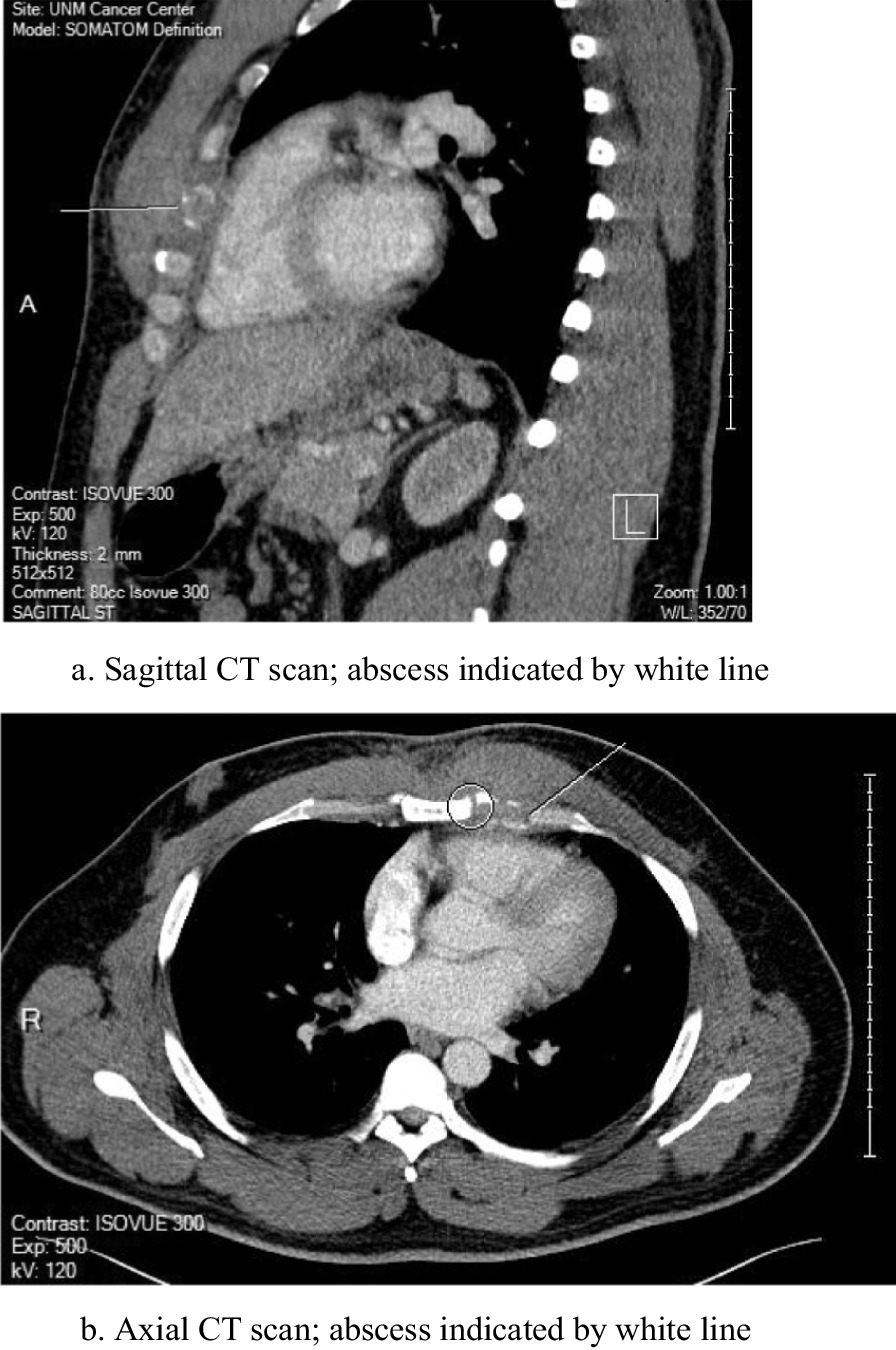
a Sagittal CT scan; abscess indicated by white line. b Axial CT scan; abscess indicated by white line
